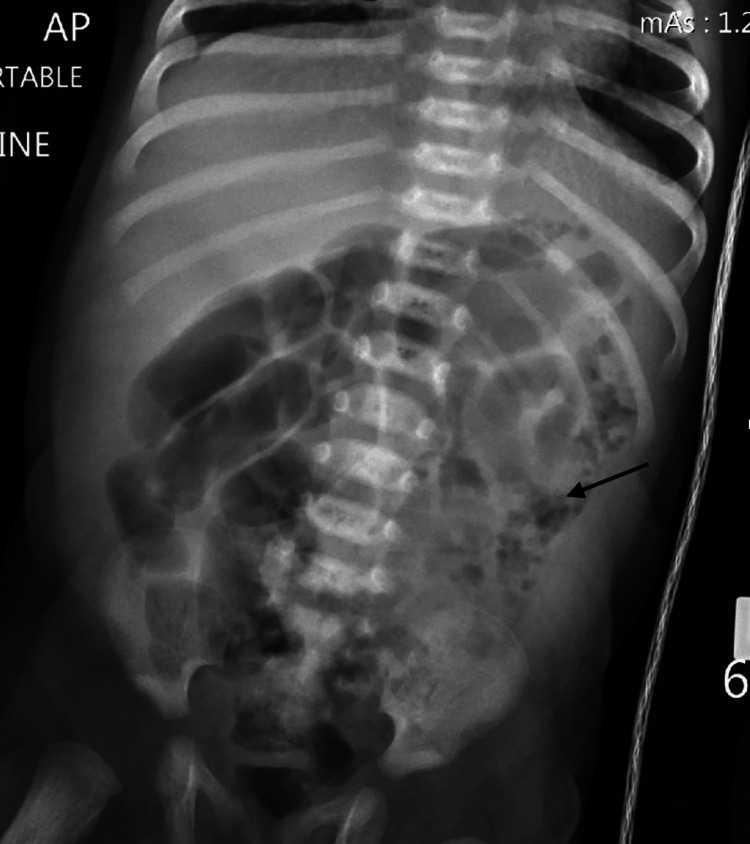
Repeat X-ray with arrow showing bubbly pattern within the splenic flexure and descending colon concerning for necrotizing enterocolitis that is more pronounced than the comparison exam.
